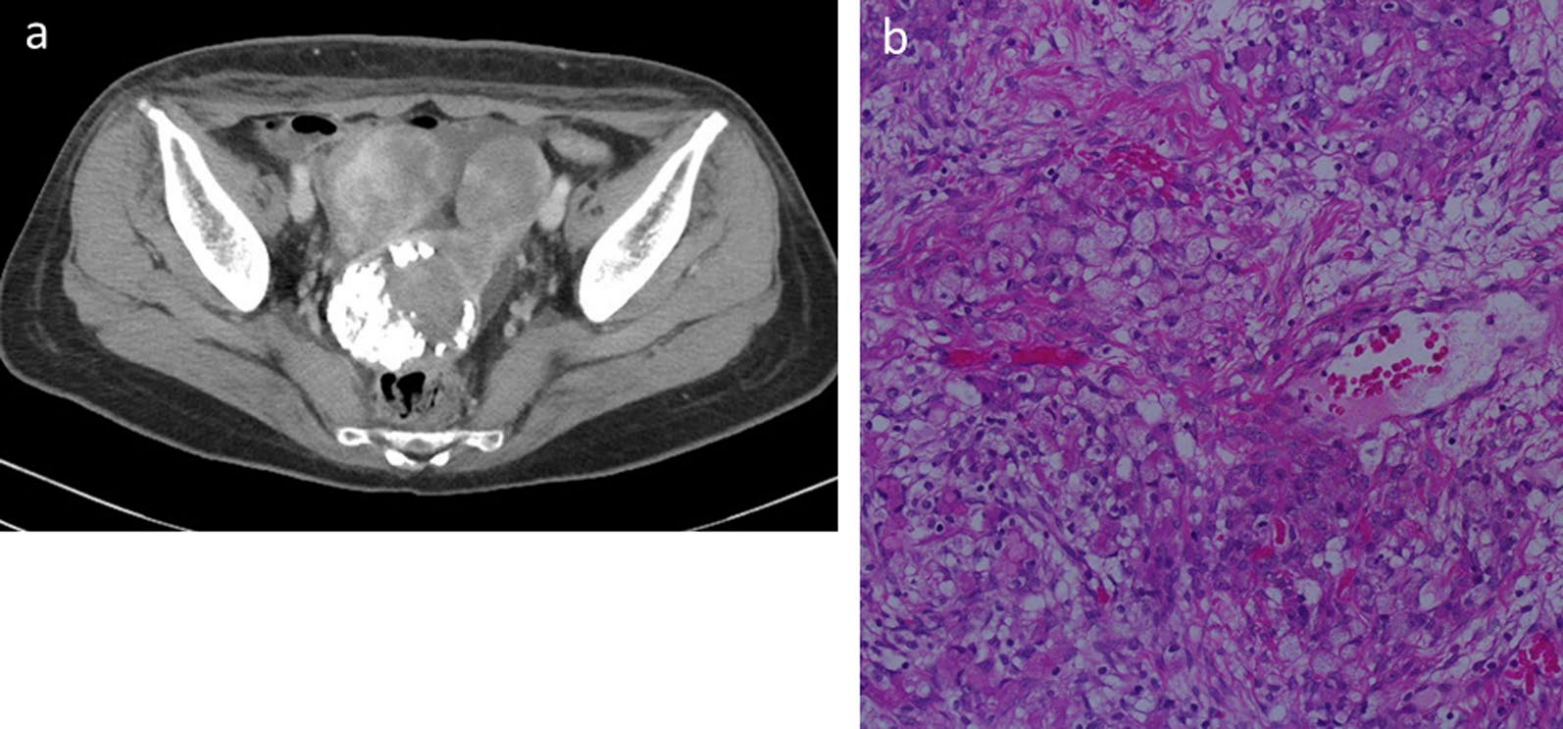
Computed tomography (CT) and pathological findings in the ovary. a Abdominal CT showed bilateral ovarian enlargement with uterine calcification due to myoma. b Pathological findings revealed signet-ring cells and a poorly differentiated adenocarcinoma (× 400 magnification)
